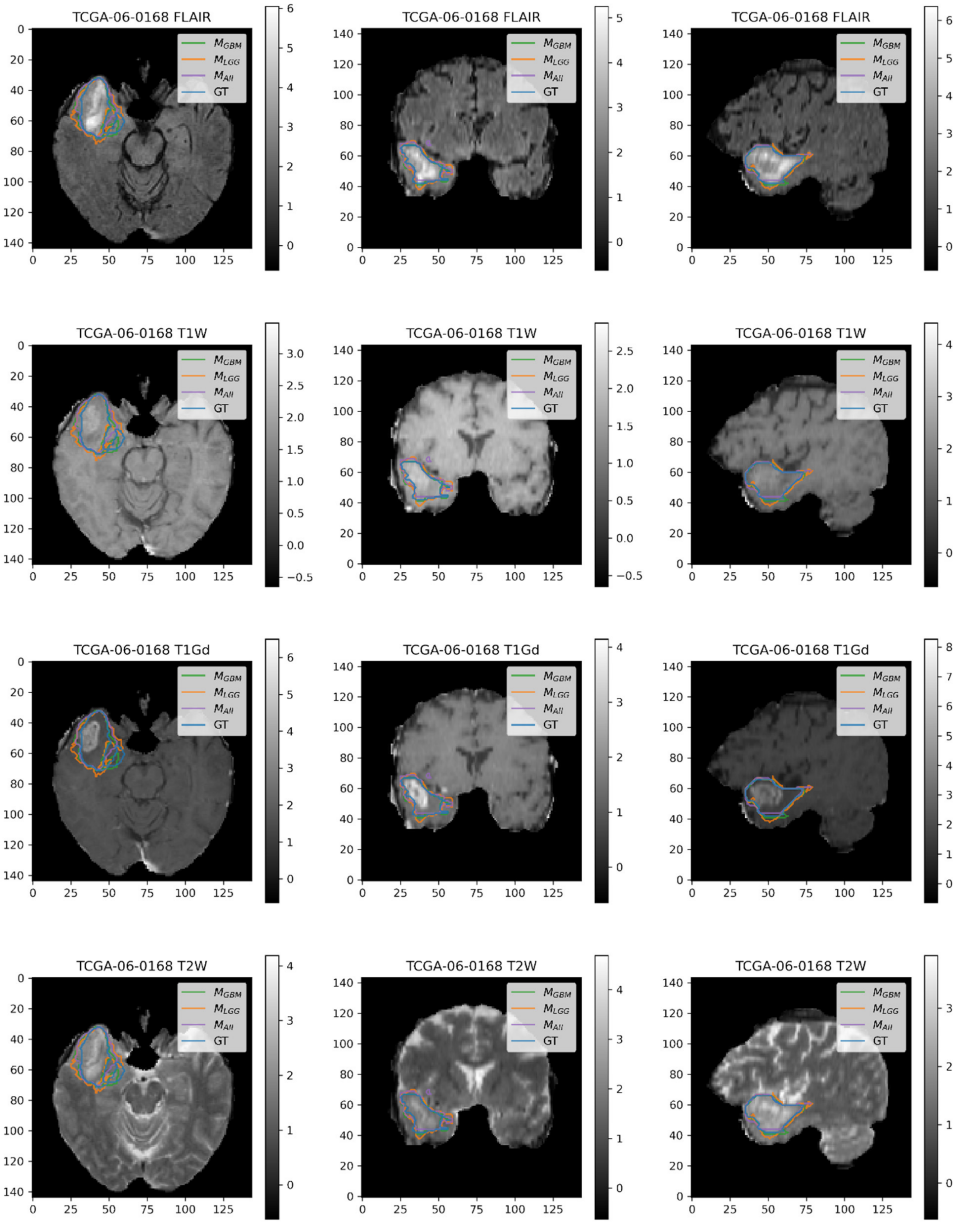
Patient TCGA-06-0168 is diagnosed with GBM in the right temporal operculum. *M*_*LGG*_ has low performance on Dice Coefficient (Dice = 0.6847) than *M*_*GBM*_ (Dice = 0.8103) and *M*_*ALL*_ (Dice = 0.8616). AUROC for all models is high despite unequal performance. The boundary of the edema on FLAIR sequence shows where *M*_*LGG*_ over-segments and *M*_*GBM*_ under-segments tumor.
